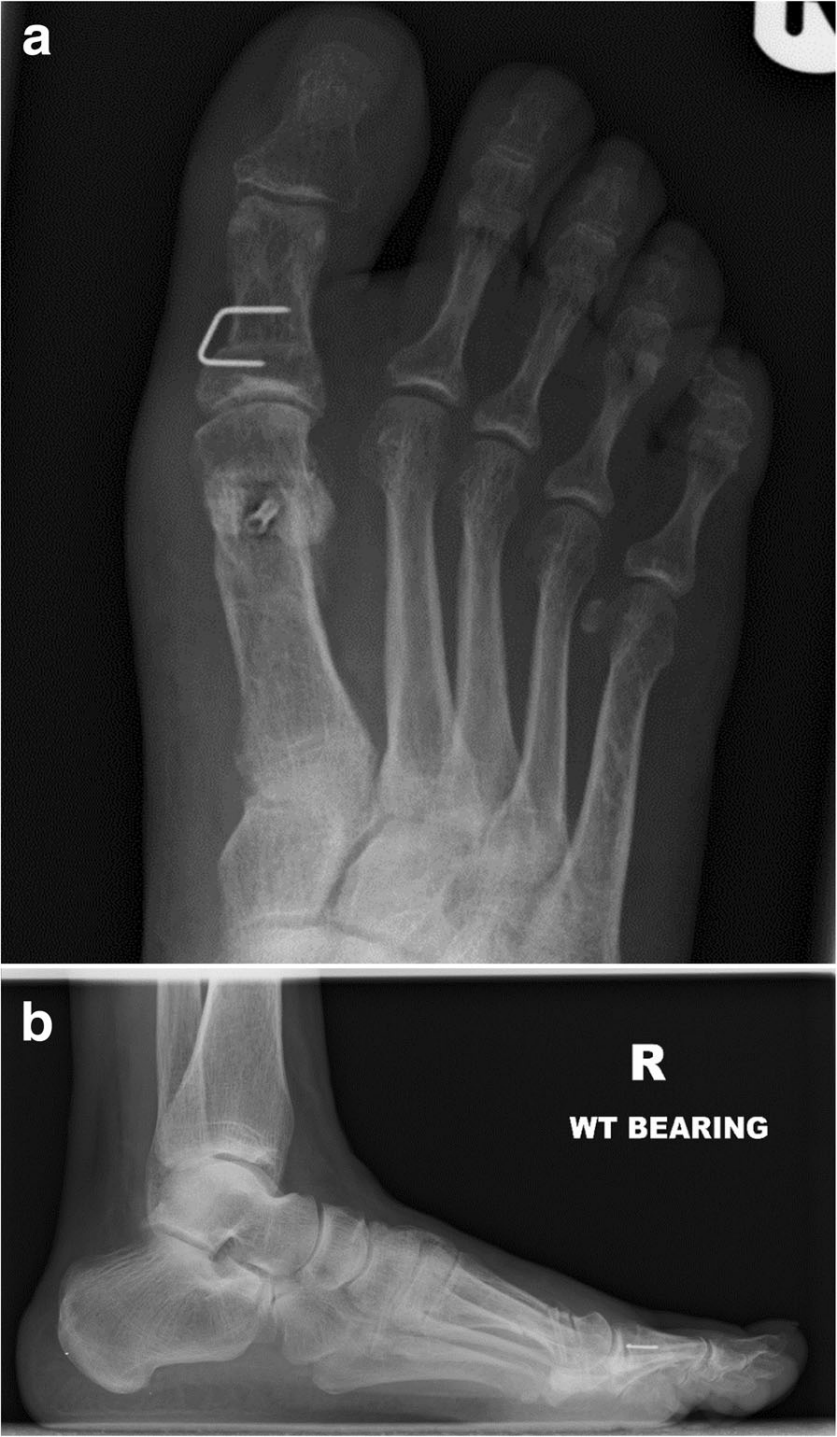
a and b Post-operative AP and lateral radiographs taken at six weeks follow up
